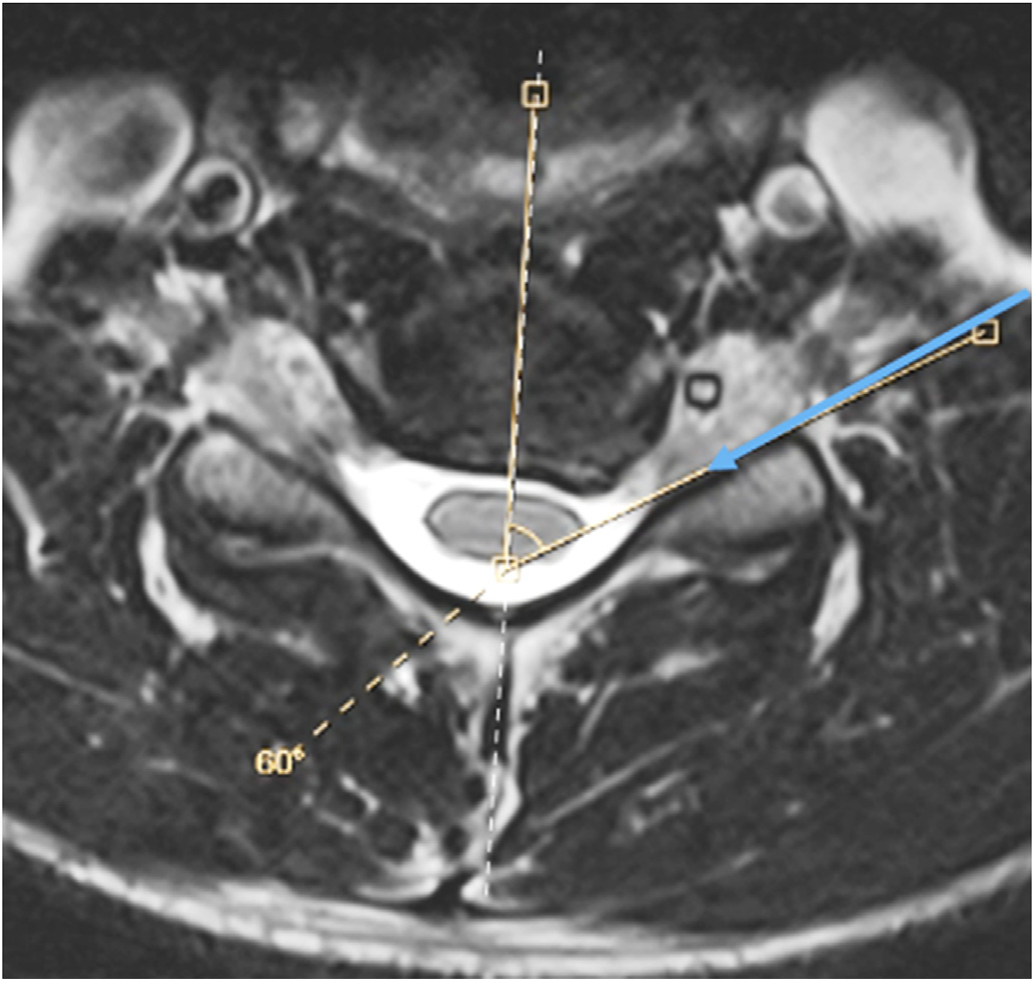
T2 Axial MRI of C5/6 with planned needle trajectory based upon measured angle of the ventral surface of the C6 superior articular process from the sagittal midline. As this SAP measured 60° on MRI. the fluoroscopic oblique angle would be preset at 60° relative to the fluoroscopic AP.
